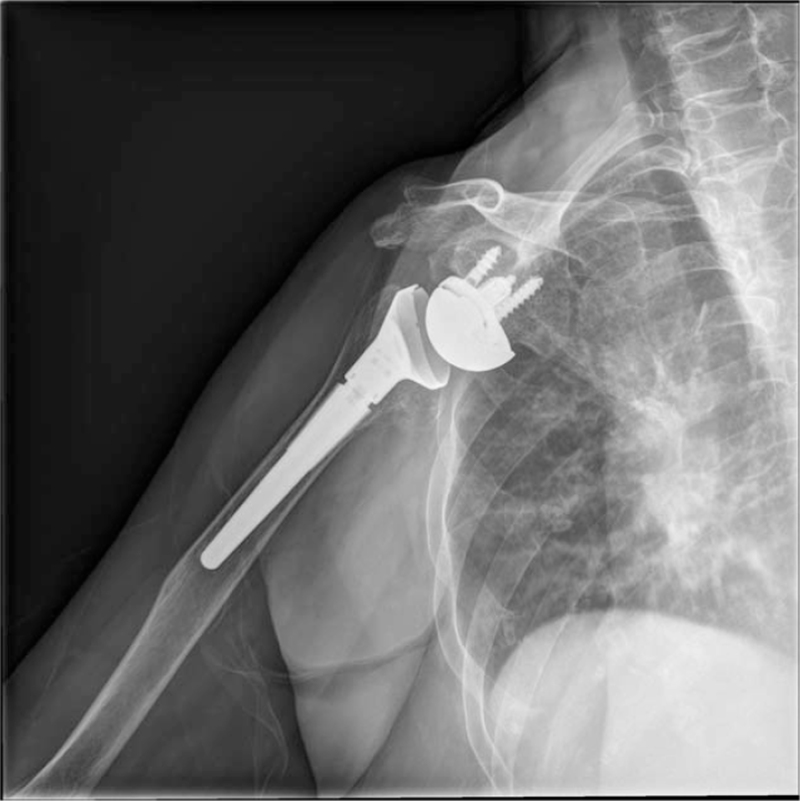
Follow-up shoulder radiograph taken 15 mo after surgery show no radiographic evidence of disease recurrence.
